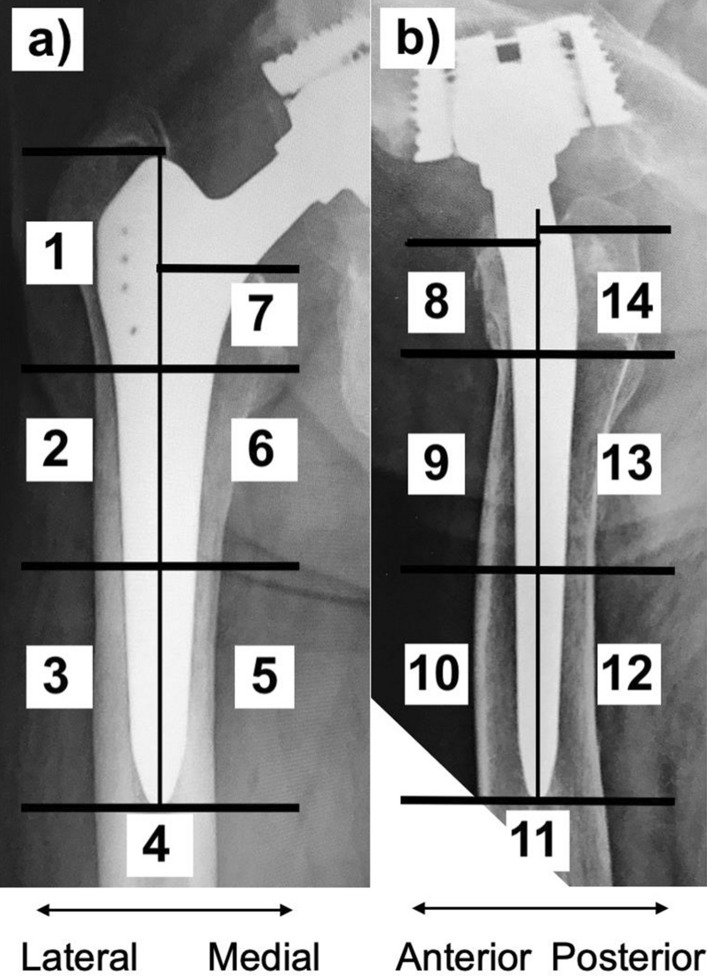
Representation scheme of Gruen zones 1–7 on an anteroposterior view (a) and 8–14 on an axial view (b) of a hip radiograph
